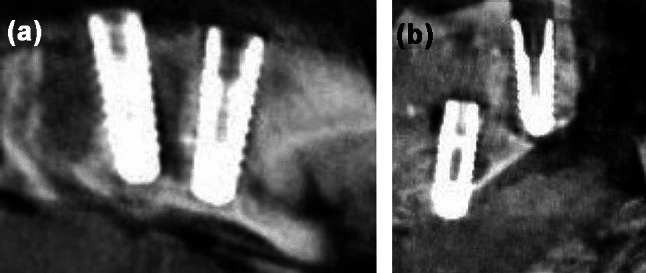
CBCT images of (a) control implants (b) implants with penetration injury (left on the image) and pilot drill injury (right on the image)
